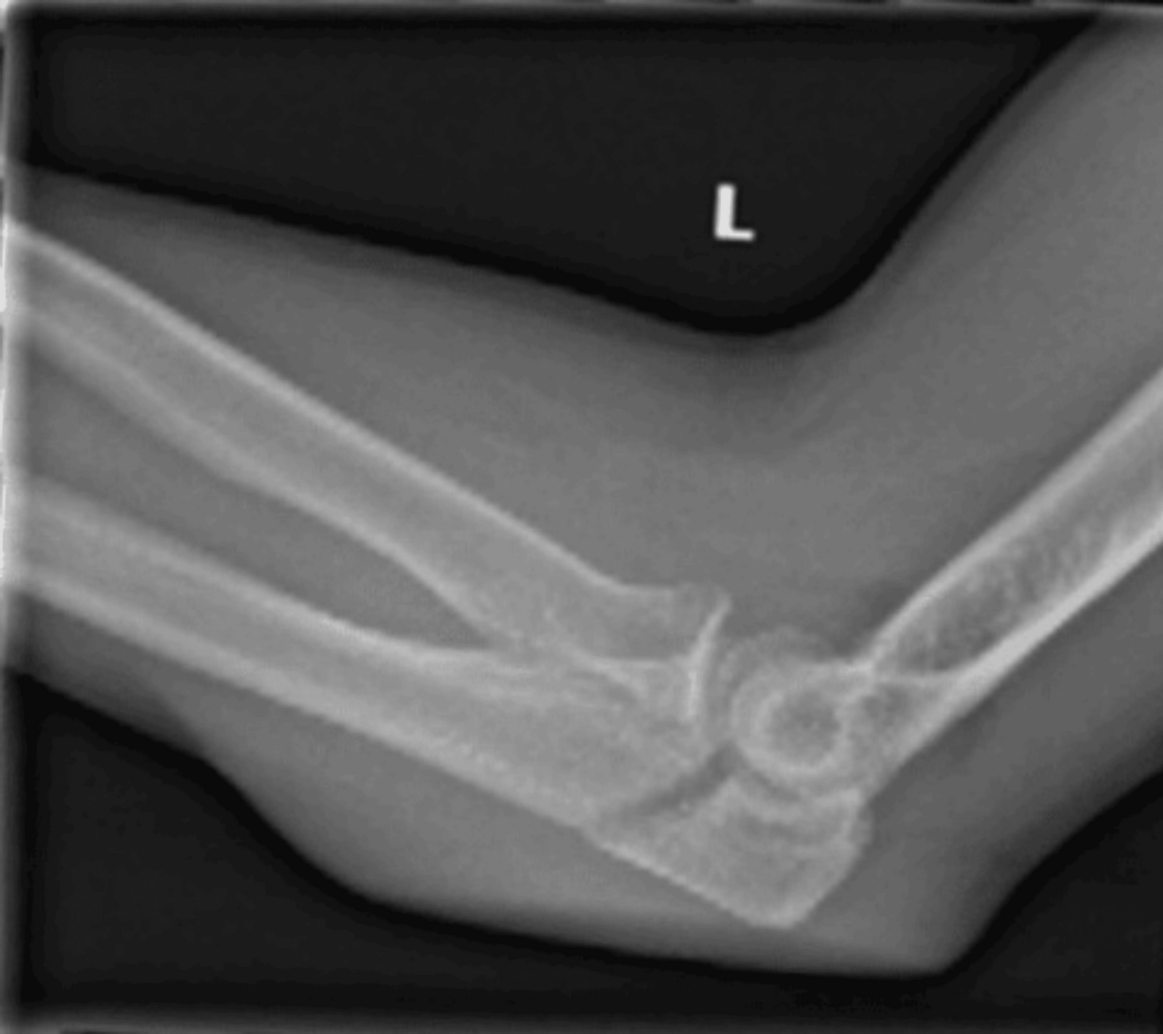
Preoperative X-ray of the olecranon Preoperative X-ray in the sagittal view of the olecranon fracture.
